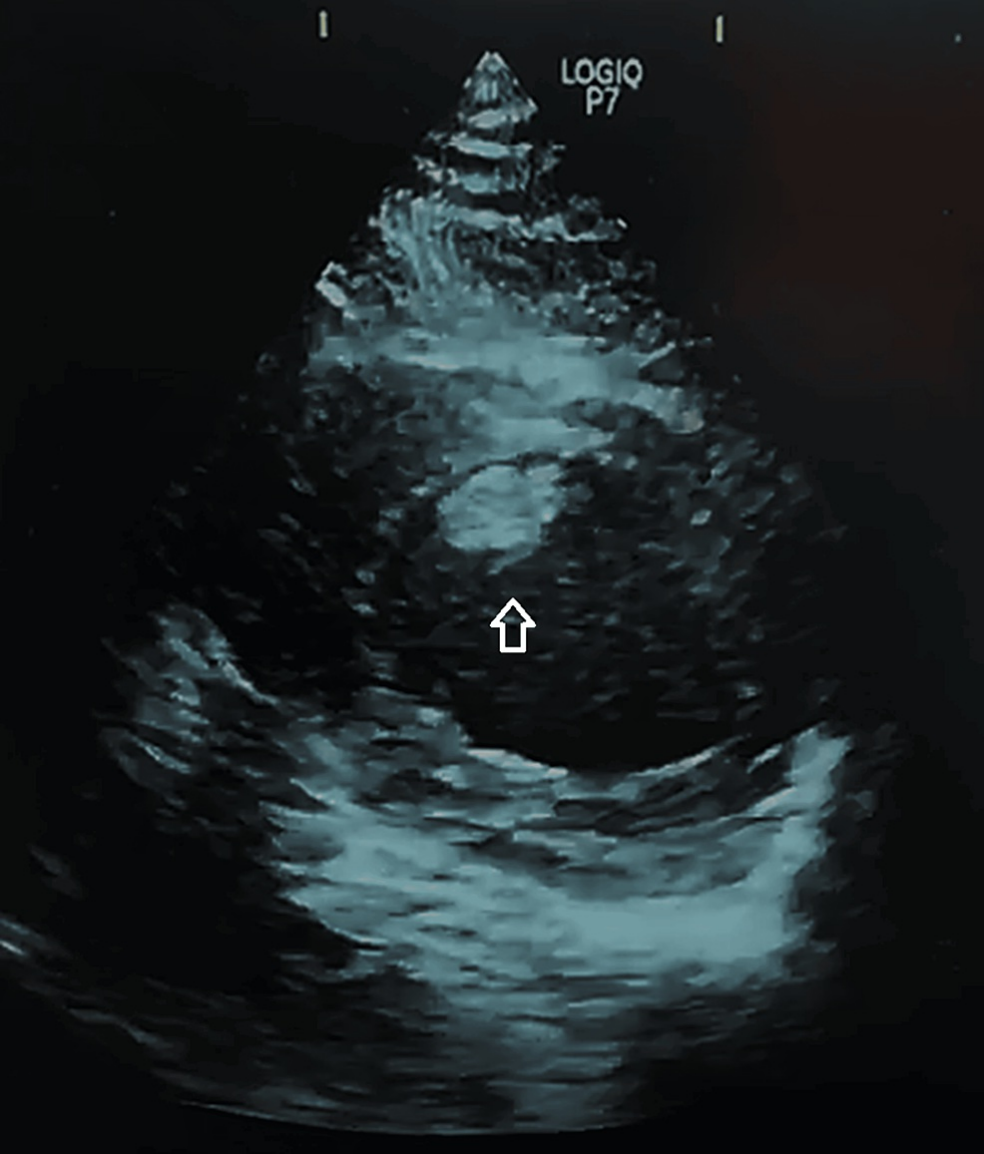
Parasternal short-axis view at the level of papillary muscles showing a large apical left ventricle thrombus (white arrow).
